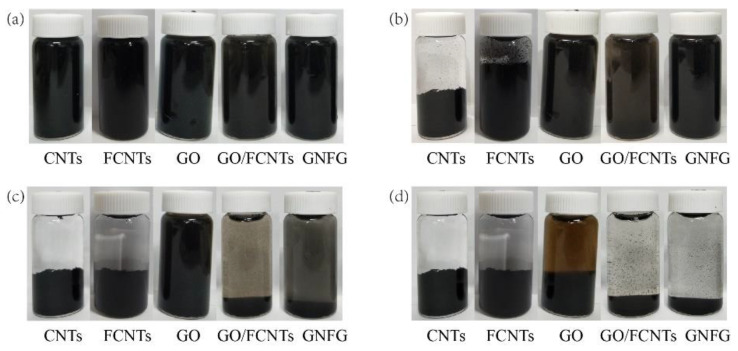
Dispersion of the nanomaterials over time after the ultrasonic treatment: (a) 0 min, (b) 10 min, (c) 6 h, and (d) 24 h.
